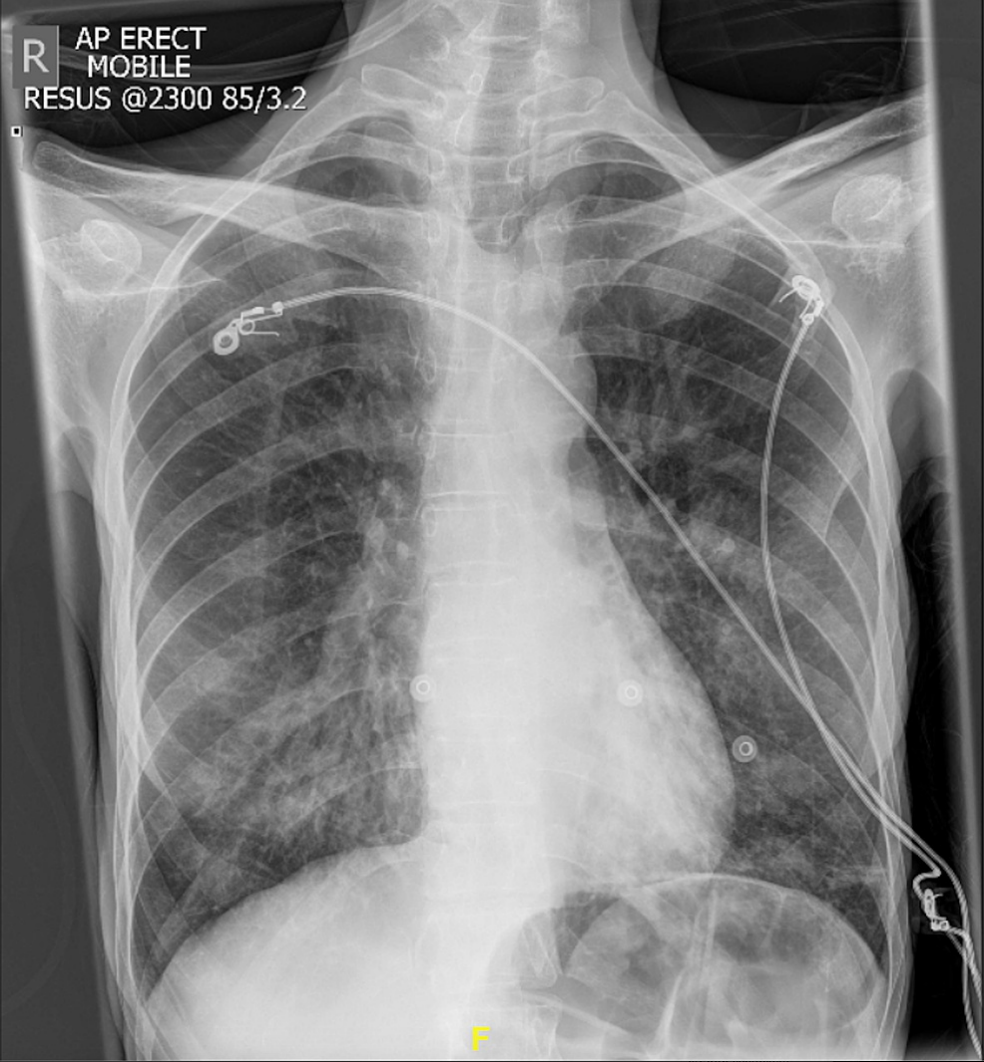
X-ray of the chest at the time of admission showing opacification in the right middle and lower zones.
